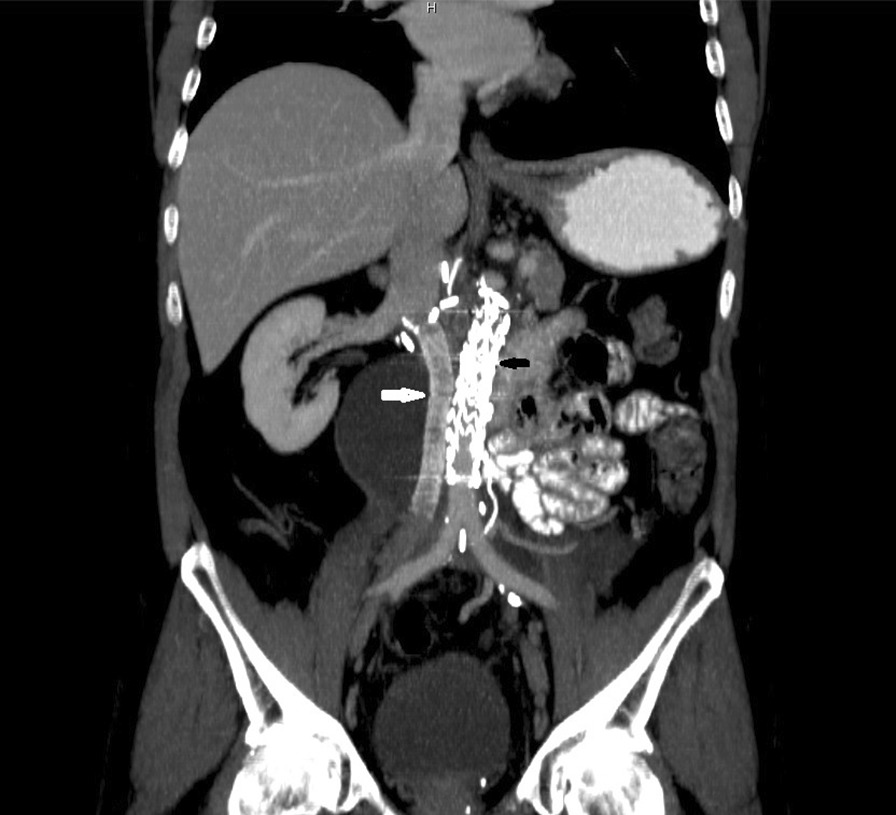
Restaging CT scan (coronal view). Aortic endograft (black arrow), venous PTFE endograft (white arrow)
